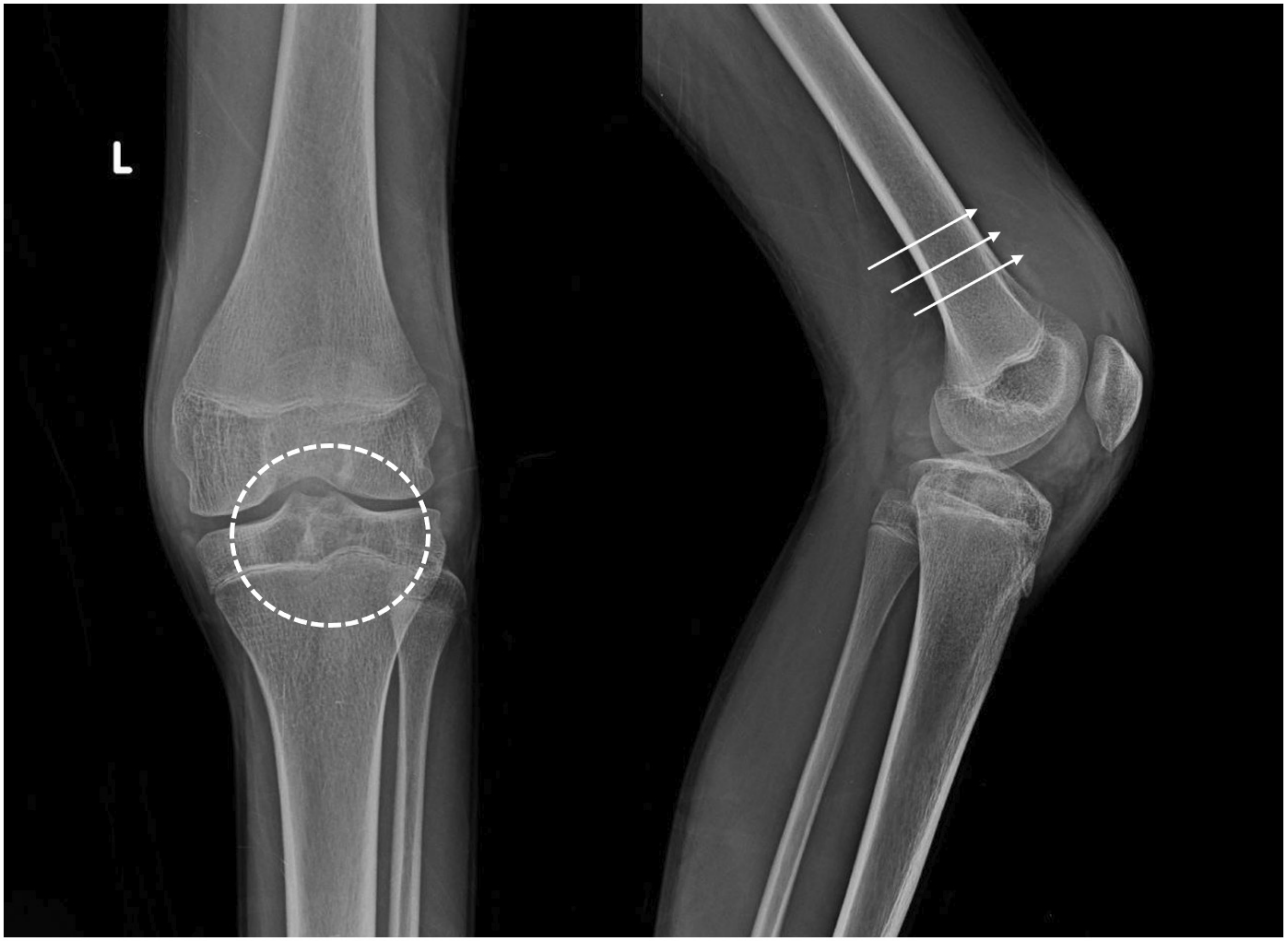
Knee radiograph showing lytic area in intercondylar eminence and along lateral tibial plateau (dotted black circle). Distension in supapatellar region with fat pad separation suggesting suprapatellar bursal fluid (white arrows).
